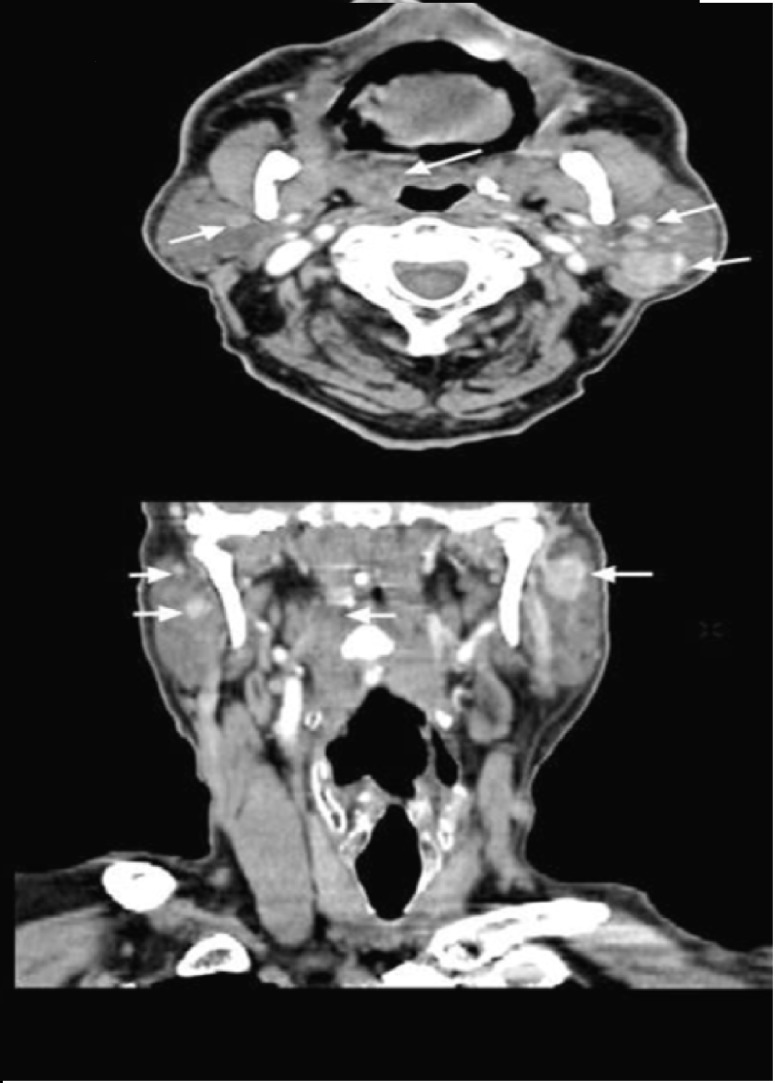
CT scan with endovenous contrast and multiplanar reconstruction: Hyperdense lesions are seen in both parotid glands, two in each gland are identified, all lesion have well-defined borders, the largest one measures 27 x 19 mm. There is asymmetry of the Waldeyer’s ring with increased volume and hyperdensity of the right side, and contralateral calcifications.
